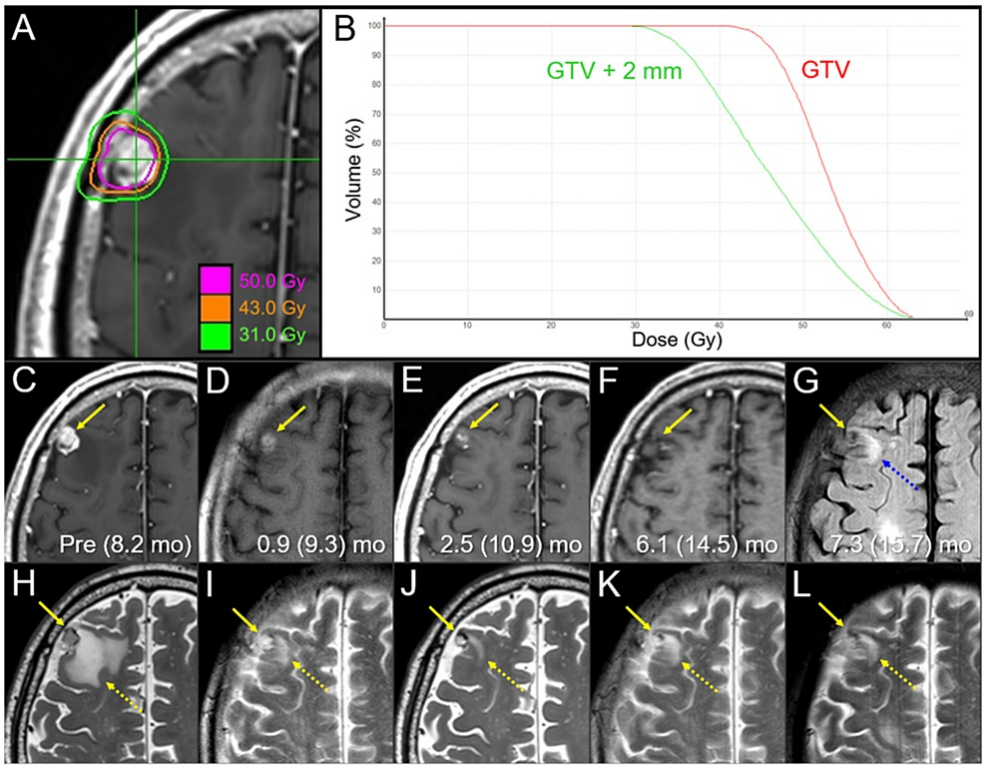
Dose distribution, dose-volume histograms, and magnetic resonance images before and after salvage 5-fr stereotactic re-radiosurgery in the right frontal lesion. The images show dose distribution (A); DVHs (B); axial CE-T1-WIs (C-F); fluid-attenuated inversion recovery (FLAIR) image (G); axial T2-WIs (H-L); at 8.2 months (mo) after the initial 8-fr SRS (six days before the salvage 5-fr re-SRS) (C, H); at 0.9 months after the re-SRS (9.3 months after the initial SRS) (D, I); at 2.5 (10.9) months (E, J); at 6.1 (14.5) months (F, K); and at 7.3 (15.7) months (G, L). (C-L) These images are at the same magnification and coordinates under co-registration and fusion. (C, H) The enhancing area of the lesion (arrow in C) exceeded the visible mass on T2-WI (arrow in H), which was associated with perilesional edema (dashed arrow in H). (D, E, I, J) From 0.9 months to 2.5 months after the re-SRS, the enhancing lesion markedly shrank, along with attenuation of the enhancing effect (arrows in D, E), the internal intensity of the visible mass on T2-WI increased (arrows in I, J), and the perilesional edema markedly decreased (yellow dashed arrows in I, J). (F, K) At 6.1 months, both the enhancing lesion (arrow in F) and the visible mass on T2-WI (arrow in K) further became obscure; however, the perilesional edema increased (yellow dashed arrow in K). (G, L) At 7.3 months, the lesion remained regressed (arrows in G, L) without expansion of the perilesional edema (yellow and blue dashed arrows in G and L, respectively). DVHs: dose-volume histograms; CE: contrast-enhanced; WI: weighted image; fr: fraction; SRS: stereotactic radiosurgery; GTV: gross tumor volume
